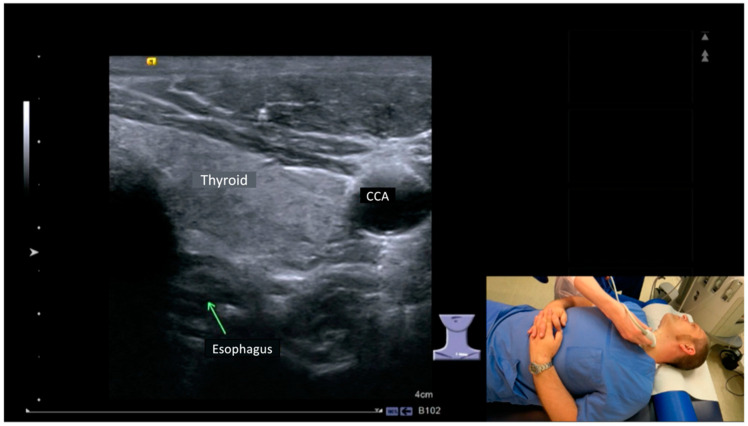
Excerpt from the step-by-step guide on how to perform a detailed HANUS examination. Ultrasound transducer and patient position were displayed in the lower right part of the screen, the standard planes were shown in the center of the screen, and the most important anatomical landmarks were labeled (ultrasound image showing the left lobe of the thyroid gland (thyroid), the esophagus, and common carotid artery (CCA)).
